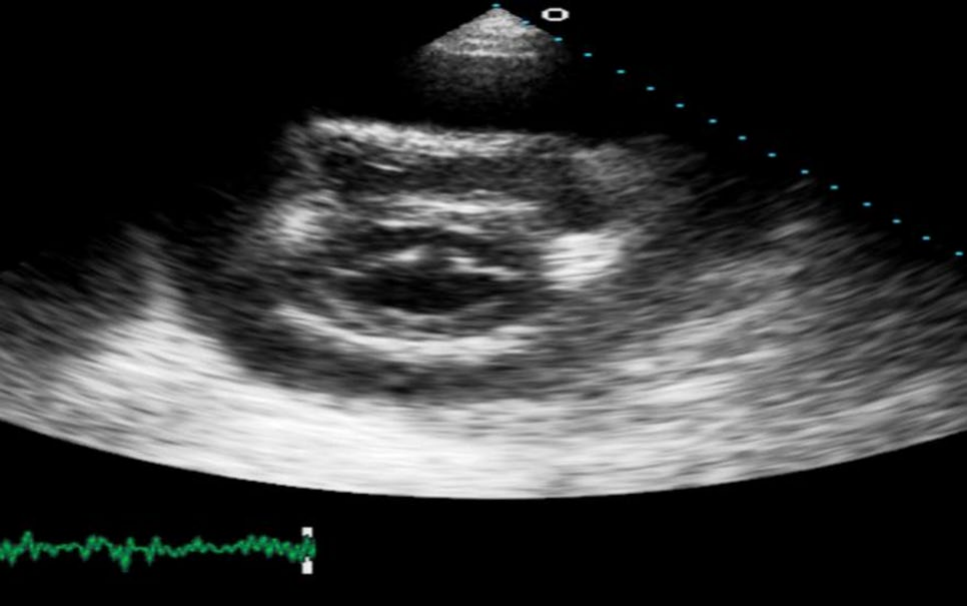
Transthoracic echocardiogram (TTE) in parasternal short axis view at level of mitral valve demonstrating diastolic collapse of right ventricular free wall secondary to large compressive pericardial effusion
